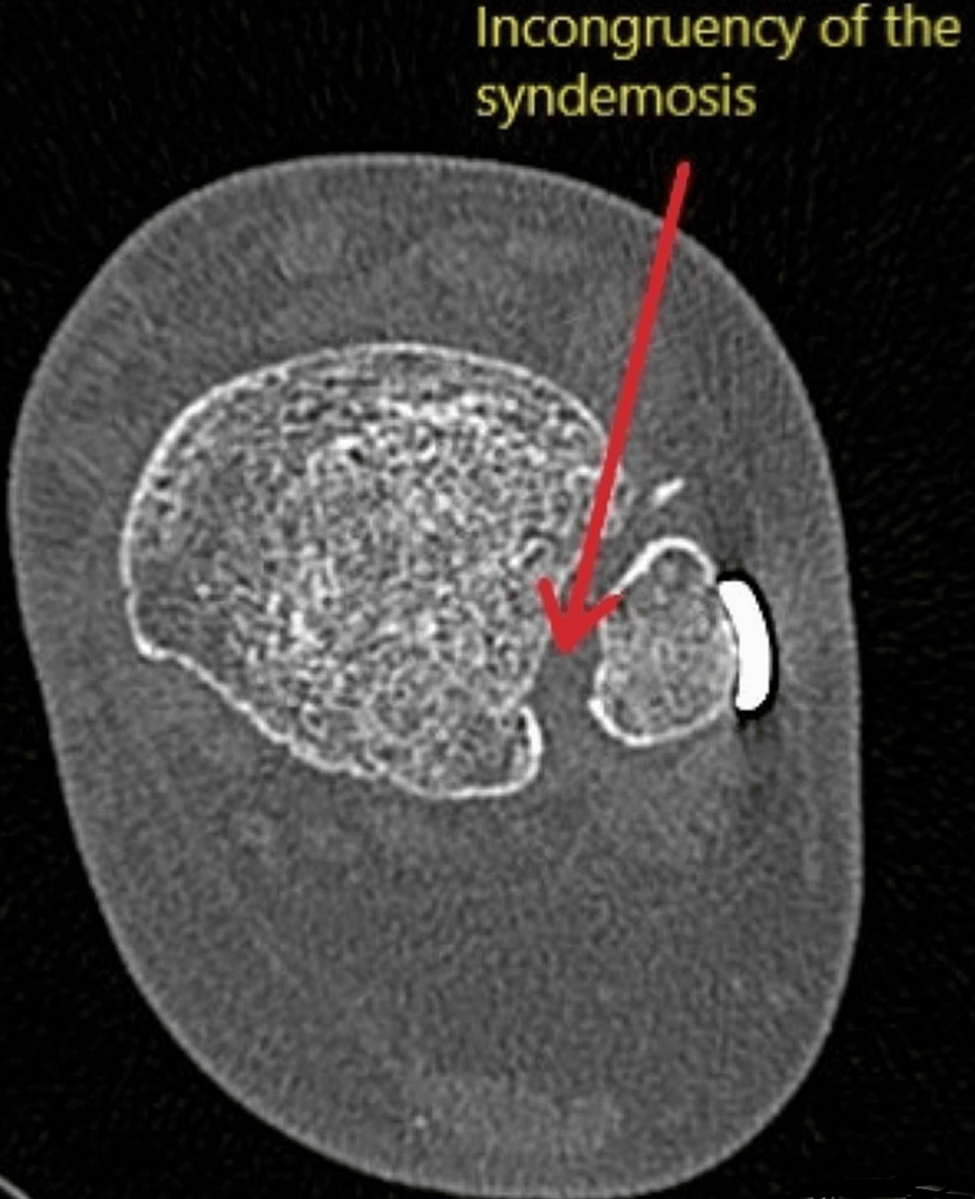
Non-contrast computed tomography (CT) scan of the ankle fixed with the suture button device showing incongruency of the syndesmosis
